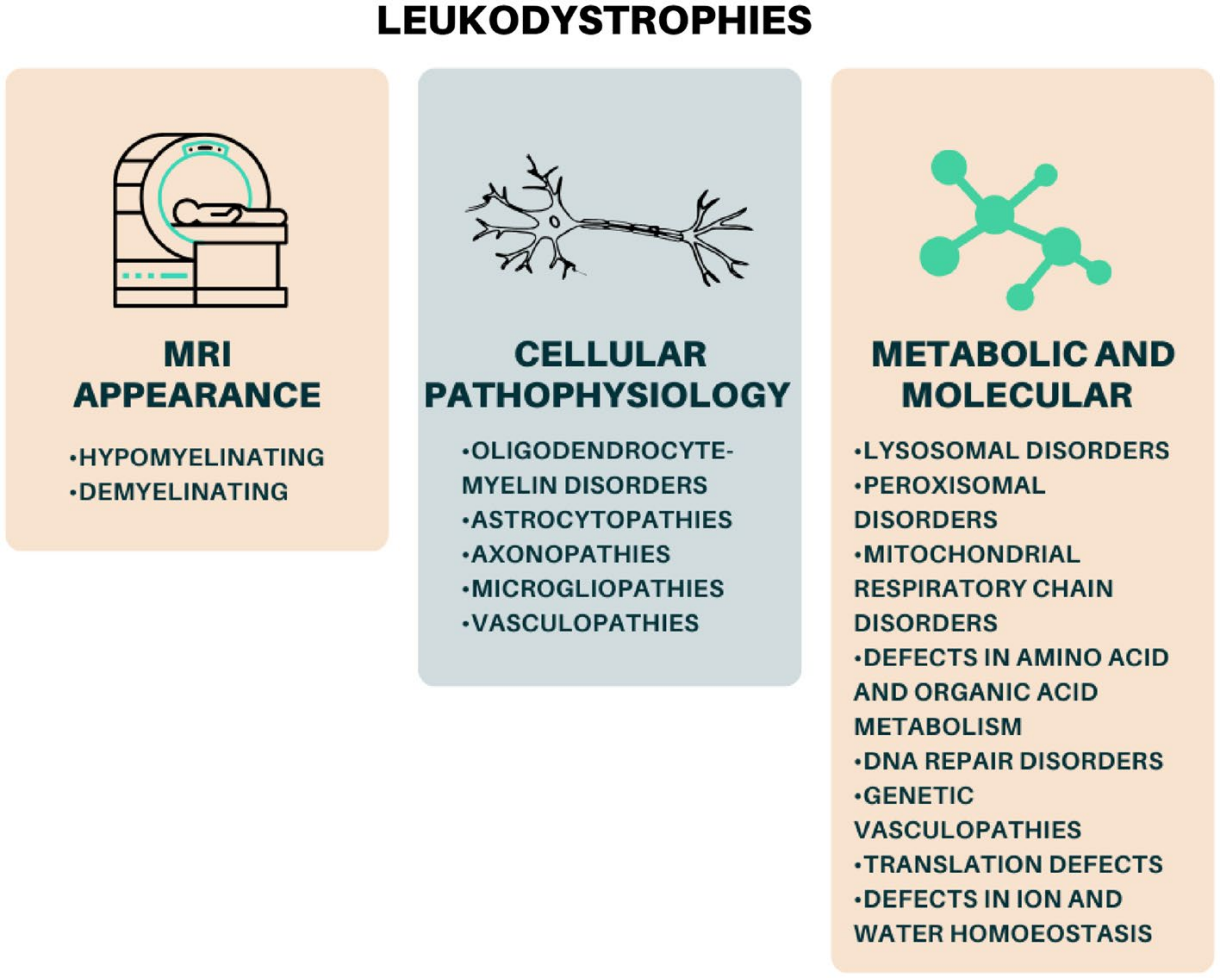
Classification of leukodystrophies based on MRI appearance, cellular pathophysiology, and metabolic and molecular mechanisms
